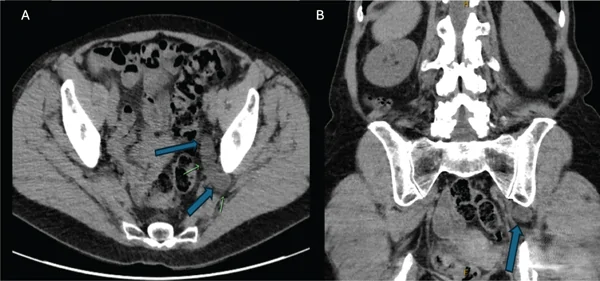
(A,B) Noncontrast (A) CT axial and (B) coronal images demonstrating the distal left ureter traced to the level of the left greater sciatic foramen through which it has herniated and abruptly tapers (blue arrows).
